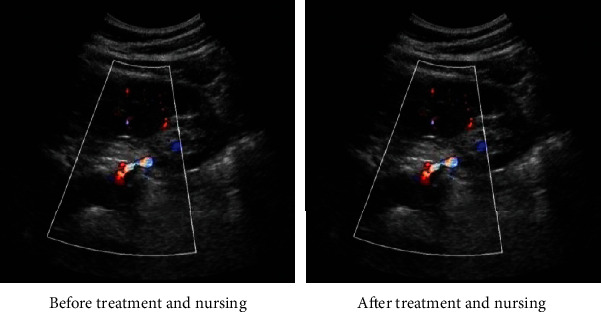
Renal ultrasound blood flow of diabetic patients under comprehensive nursing.
